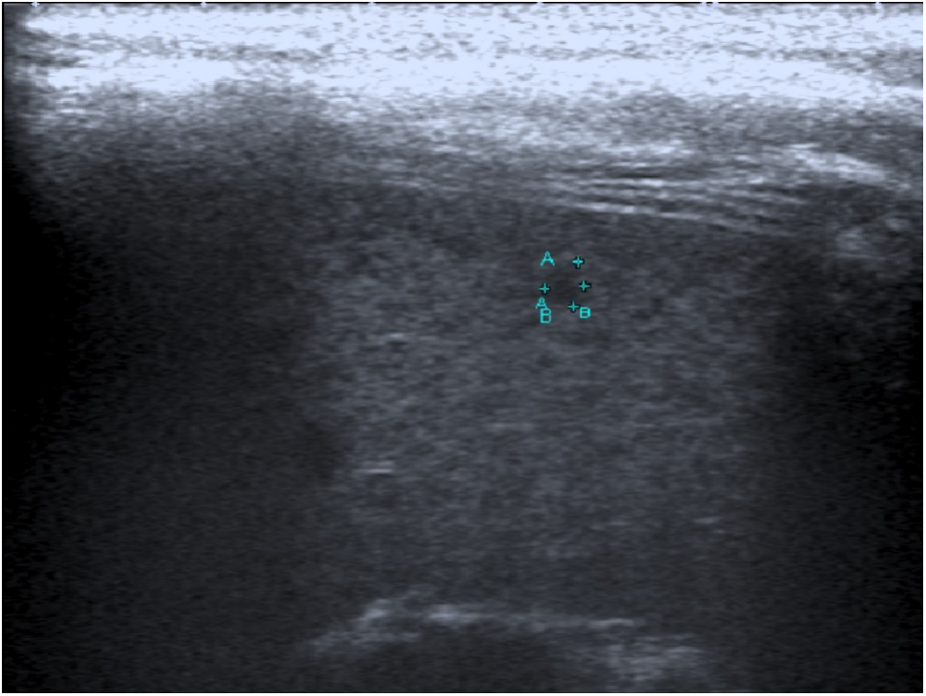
Image of an 11-year-old girl with prolonged fever and multiple small splenic abscesses who was diagnosed with childhood-onset SLE. Abdominal sonogram showing a 0.3 cm hypoechoic splenic lesion.
